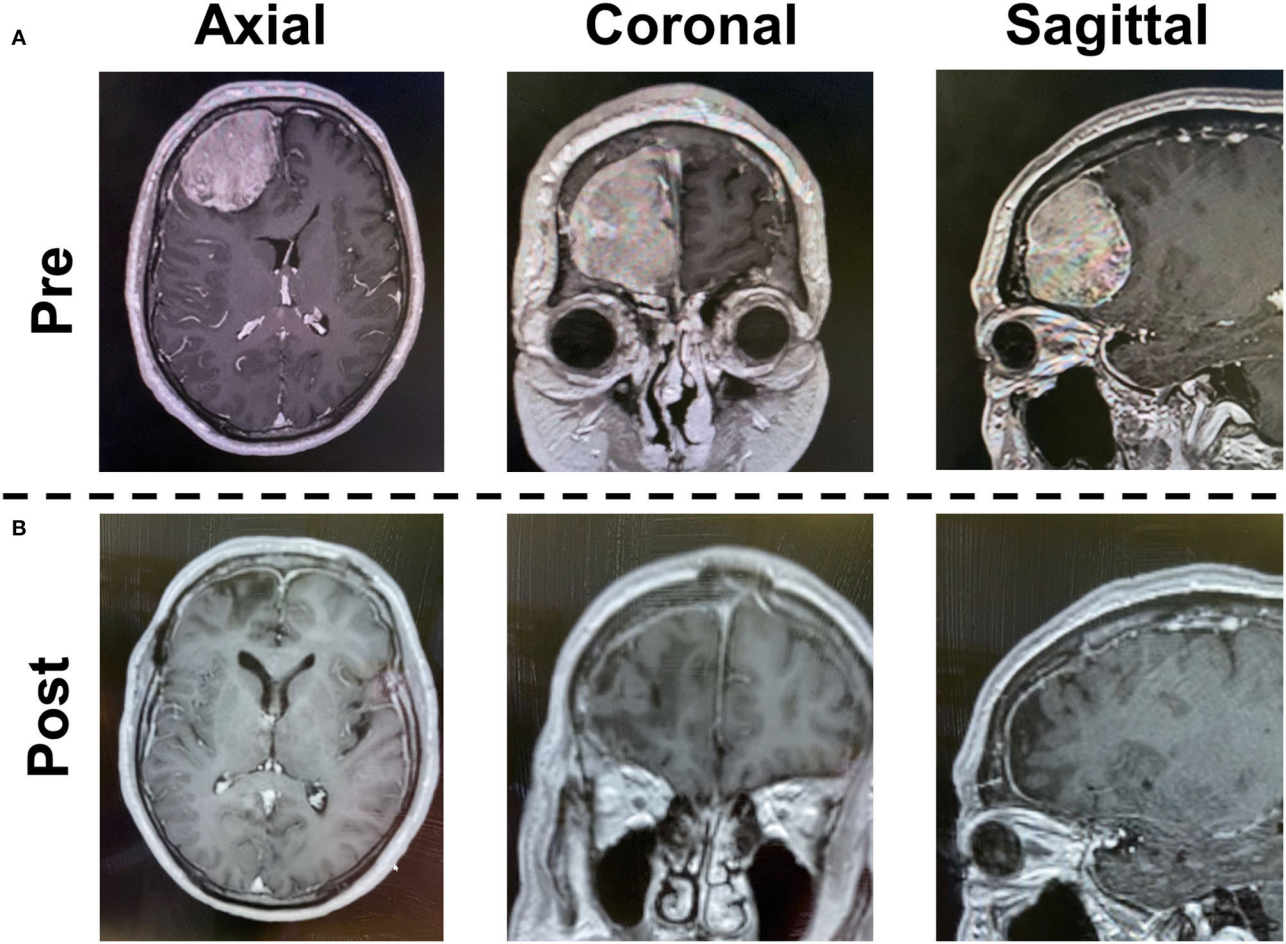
Case presentation. Preoperative (A) and postoperative (B) gadolinium-enhanced T1-weighted imagings are shown.
